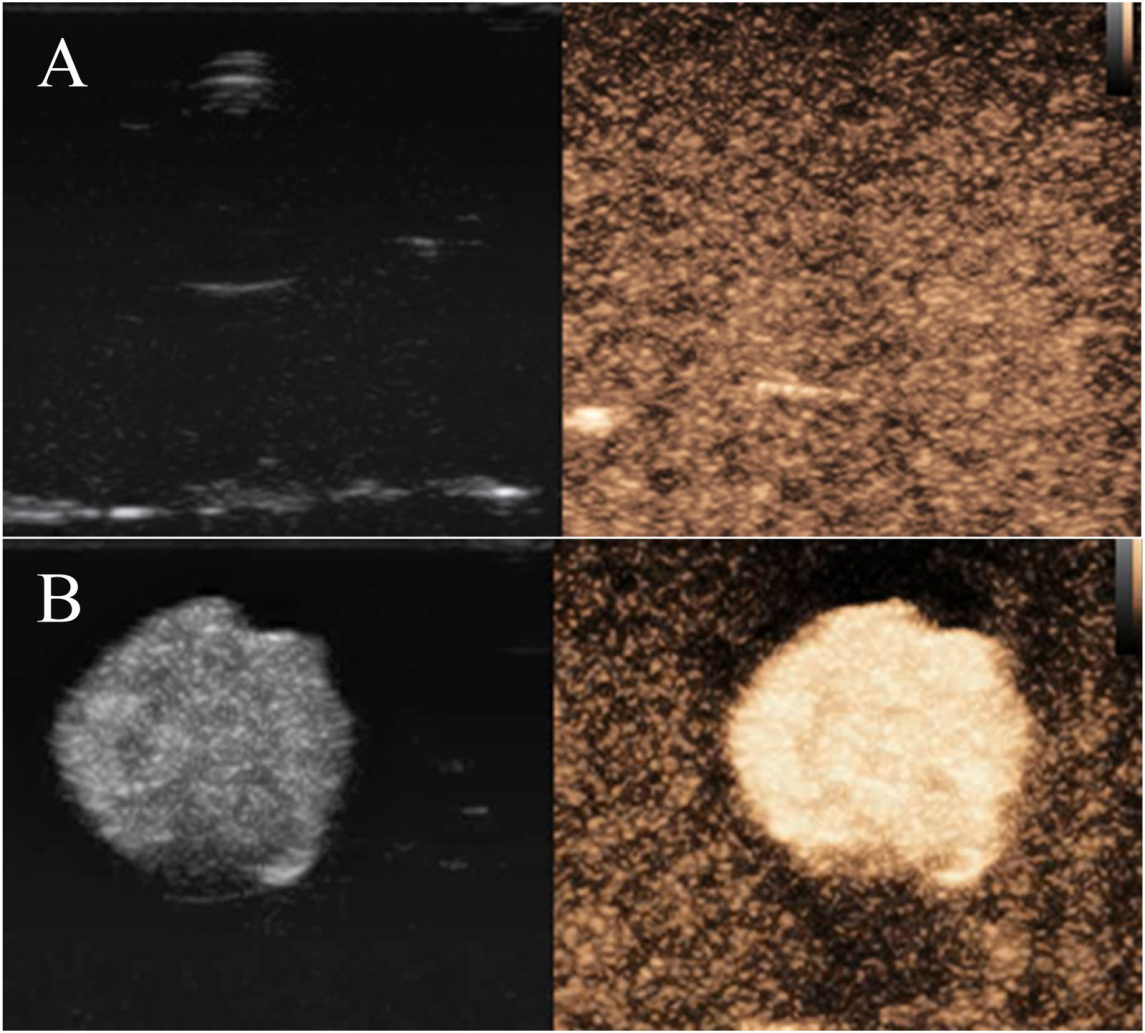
(A) Contrast-enhanced ultrasonography of PBS solution in agar block model; (B) Contrast-enhanced ultrasonography of FA-TNBs solution in the agar block model.
